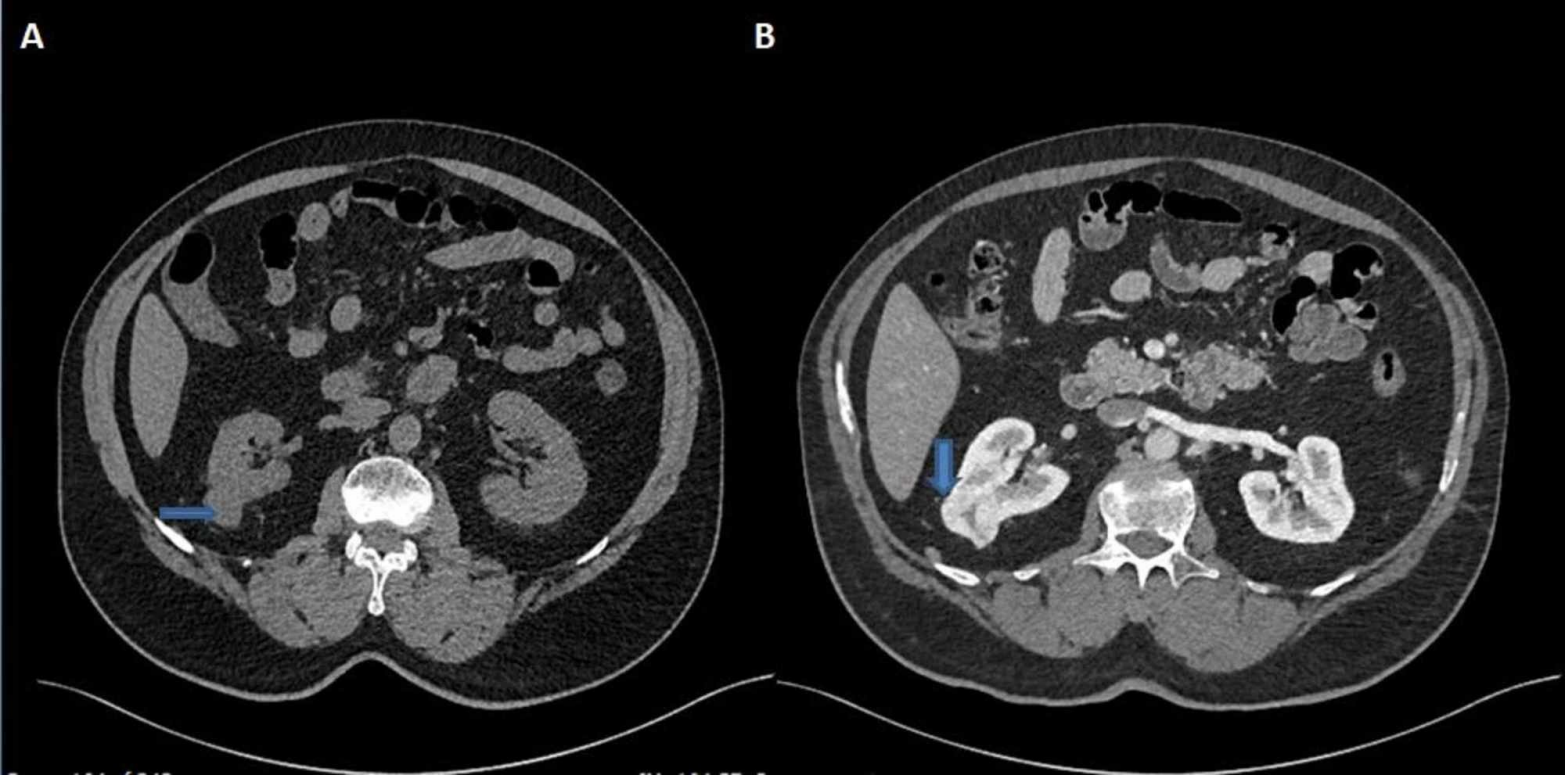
Axial sections of CT of the abdomen and pelvis It reveals an exophytic mass lesion measuring 27 mm in size in the interpolar region of the right kidney (A). It is demonstrating contrast enhancement with a central non-enhancing area (B).
